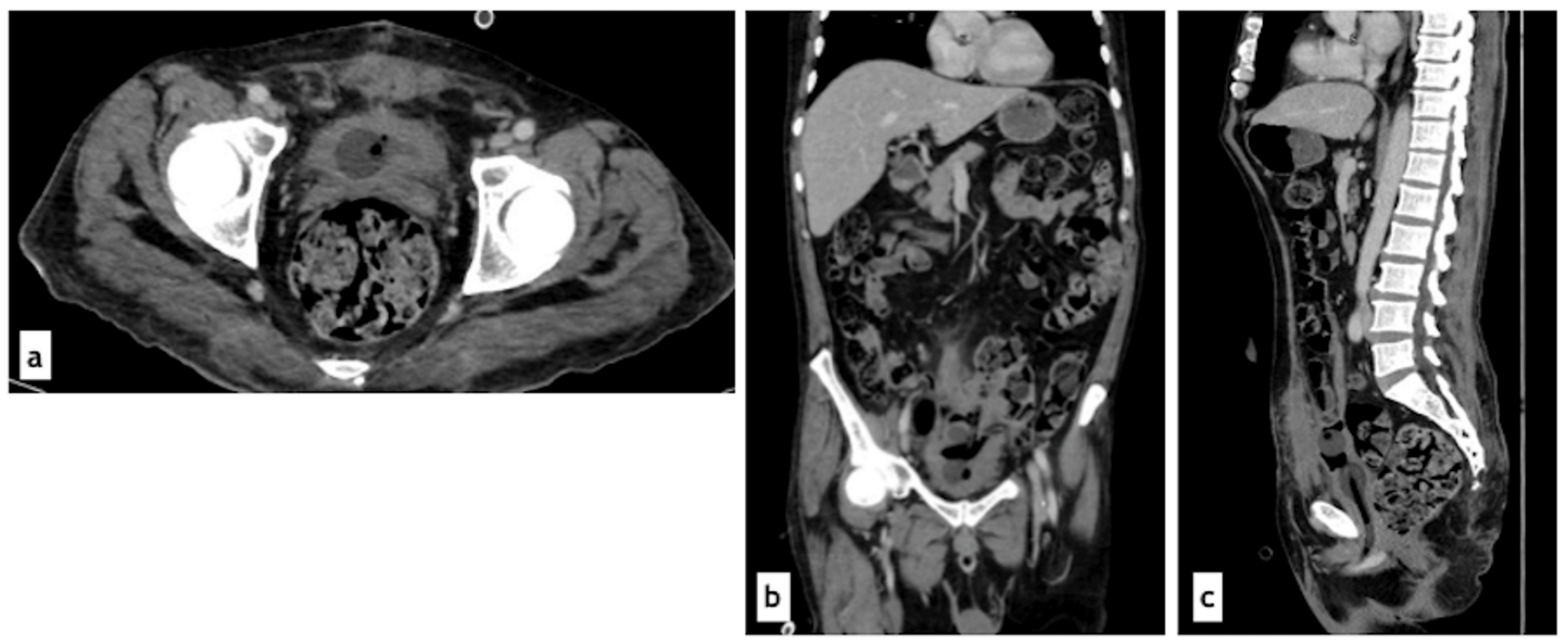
Postoperative abdominal computed tomography scan. Contrast-enhanced abdominal computed tomography, venous phase: (a) Axial view showing a partially collapsed bladder with a Foley catheter balloon inside. (b) Coronal view showing both urinary catheter balloons (cystostomy and transurethral) within the bladder. (c) Sagittal view demonstrating the same findings as (a) and (b).
